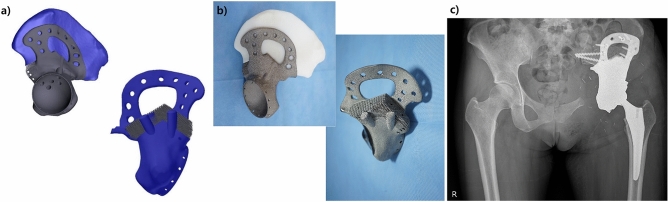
Pelvic implant manufactured by metal 3D printing for a 35-year-old woman who received surgery for Ewing sarcoma of the left pelvis. (a) Designs and (b) photographs revealed that the pelvic implant has both solid and lattice structures. (c) A postoperative plain radiograph of the applied pelvic implant.
